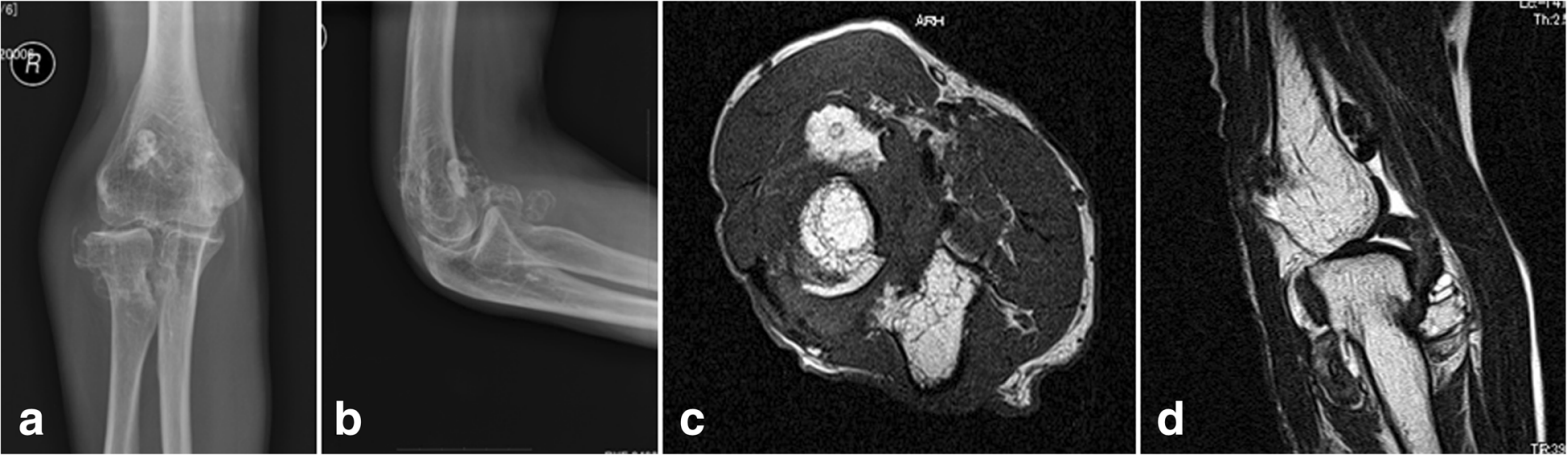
Preoperative plain radiographs and plain magnetic resonance images of the right elbow. a Anteroposterior view and b lateral view of plain radiographs. Osteoarthritic change is visible with ectopic calcifications and ossicles anteriorly, posteriorly, and laterally in the elbow. c Axial view and d sagittal view of the plain magnetic resonance images. A mass lesion is visible around the radial head and neck, anterior to the humerus, and in the olecranon fossa, with heterogeneous intensity on T1-weighted and T2-weighted images
